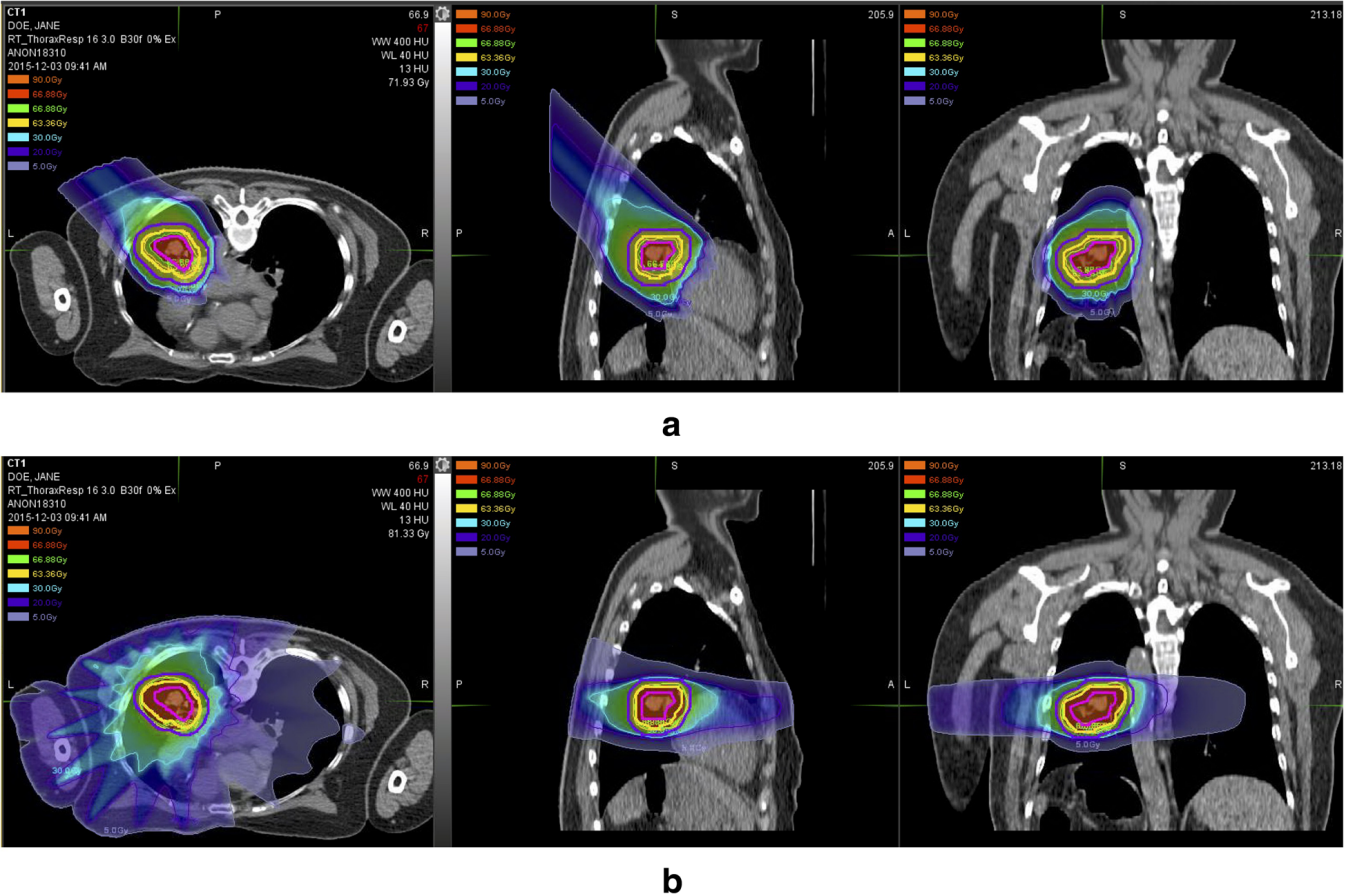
Typical dose distribution of CIRT (pencial beam scannning) (a) vs. X-ray based radiation therapy (RapidARC®) (b). CIRT significantly reduced the doses to the contralateral lung, heart, spinal cord, and the volume of low-dose area as compared to X-ray based arc therapy
